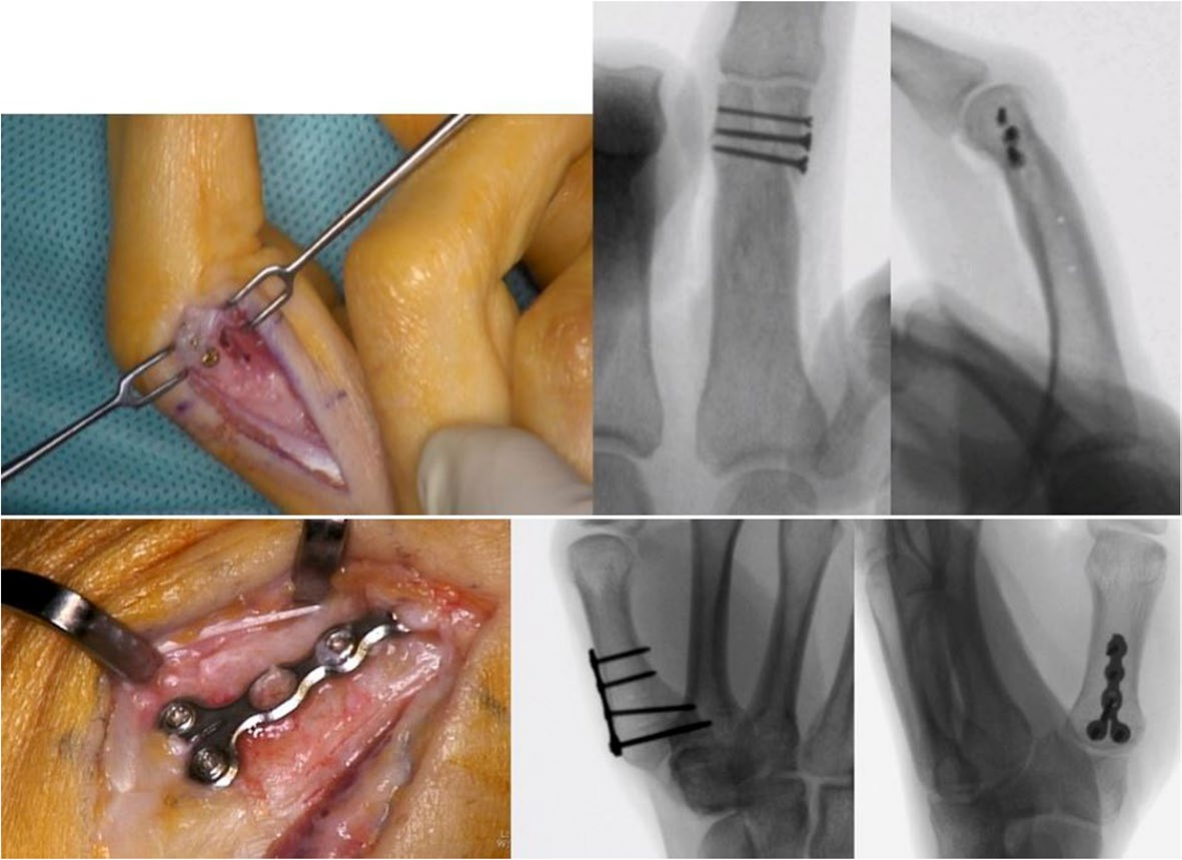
Top: Left: Intraoperative screw fixation of reposition. Right: Image intensified radiographic examination after reduction and fixation; bottom: Left: Intraoperative plate fixation of reposition. Right: Image intensified radiographic examination after reduction and fixation
